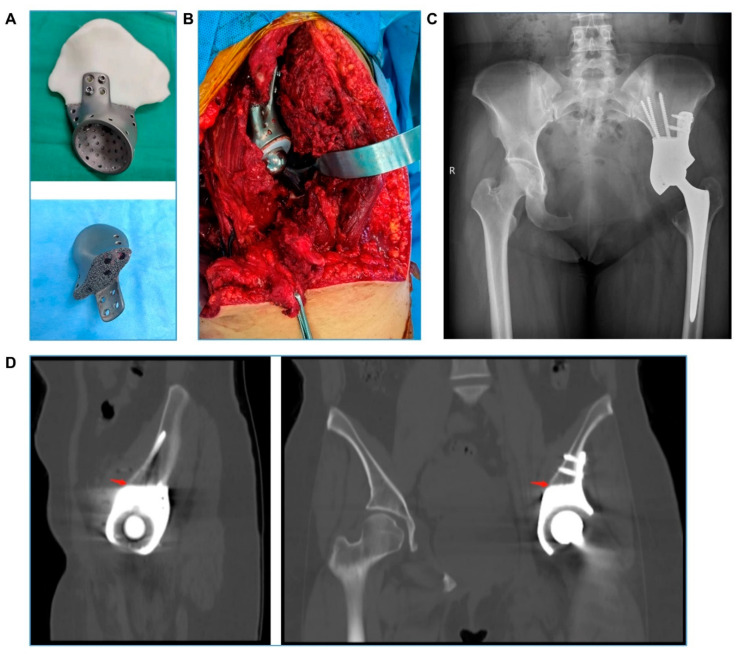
Case 3. Intra- and post-operative images. (A) Finished 3D-printed prosthesis: The porous side is clearly shown. (B) The 3D-printed prosthesis is installed and fixed. (C) X-ray shows a good implantation effect at the visit 3 months post operation. (D) CT shows good bone ingrowth and fusion at the prosthesis and bone interface (red arrow) at the visit 10 months post operation.
